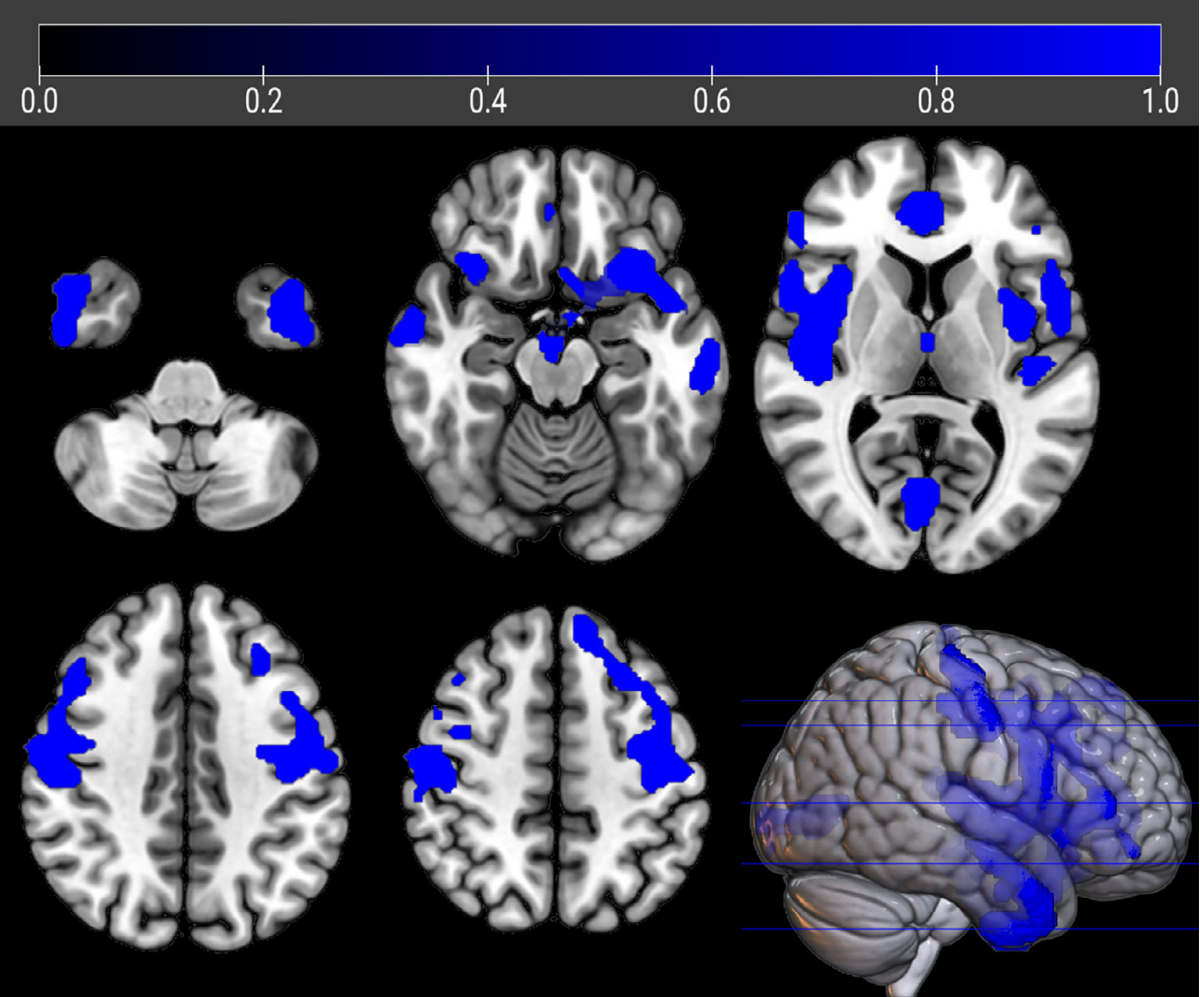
The regions showing a statistically significant relative hypometabolism in sALS patients as compared to *FUS*‐ALS subjects are marked in blue and are reported on axial sections of a brain MRI template and on the brain surface of a glass brain rendering (bottom right).
